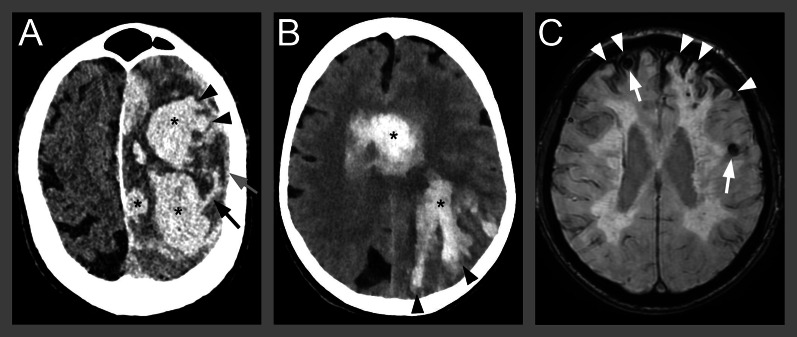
Cerebral amyloid angiopathy imaging features. Examples of imaging features of cerebral amyloid angiopathy (CAA) on CT and MRI. CT imaging predictors of CAA (panel A and B) showing ICH with multifocal components (black asterisks), finger-like projections (black arrowheads), subarachnoid hemorrhage component (black arrow) and subdural hemorrhage component (gray arrow). MRI of cerebral amyloid angiopathy (panel C) showing multiple lobar cerebral microbleeds (white arrows) and cortical superficial siderosis (white arrowheads)
